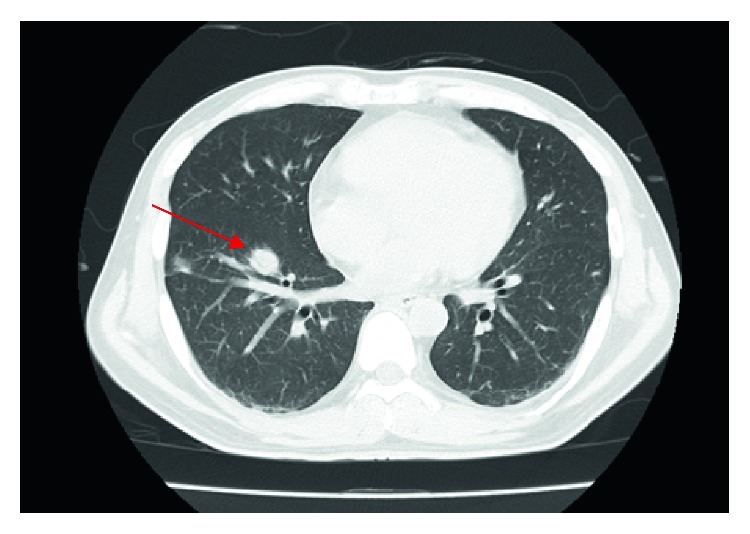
The chest computed tomography on the 1^st^ day showing multiple nodules (red arrow).
